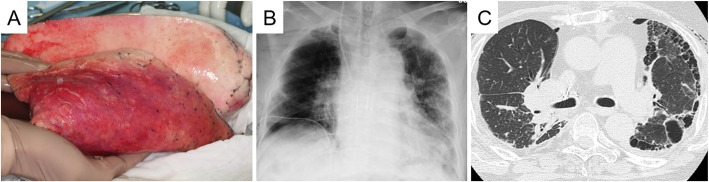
a Photograph of the left graft. Broad edematous lesions became apparent in the left lower lobe of the graft lung after flushing. b Chest X-ray and c chest computed tomographic image on postoperative day 7. The chest X-ray and chest computed tomographic images obtained on postoperative day 7 revealed the prompt amelioration of the edematous changes in the donor lower lobe in the reverse position and the adaptation of the left donor lung in the recipient right chest cavity without the development of atelectasis
